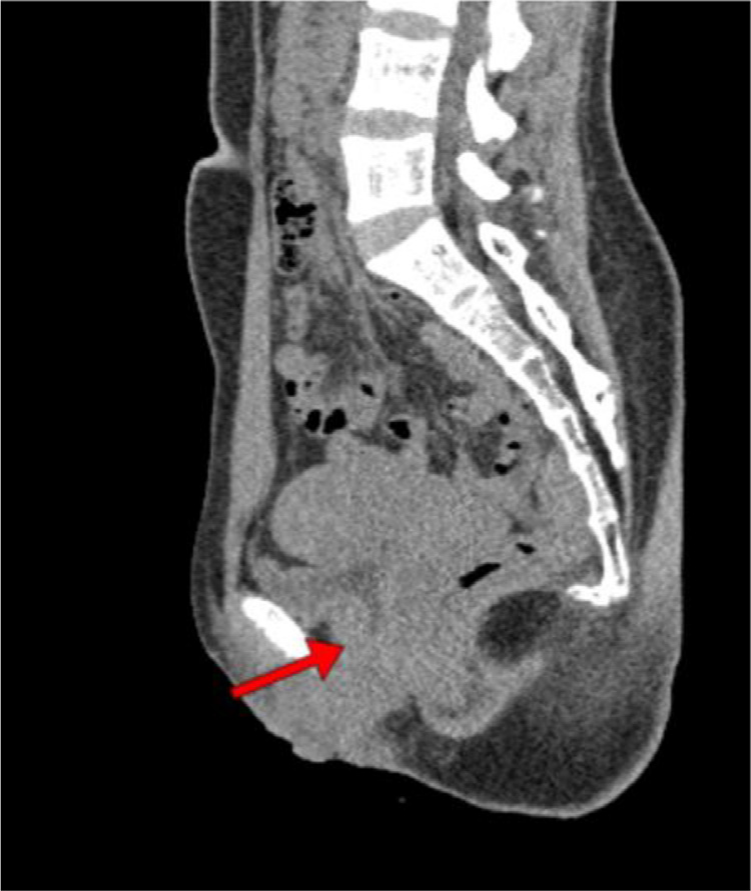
Noncontrast abdominal CT scan.
